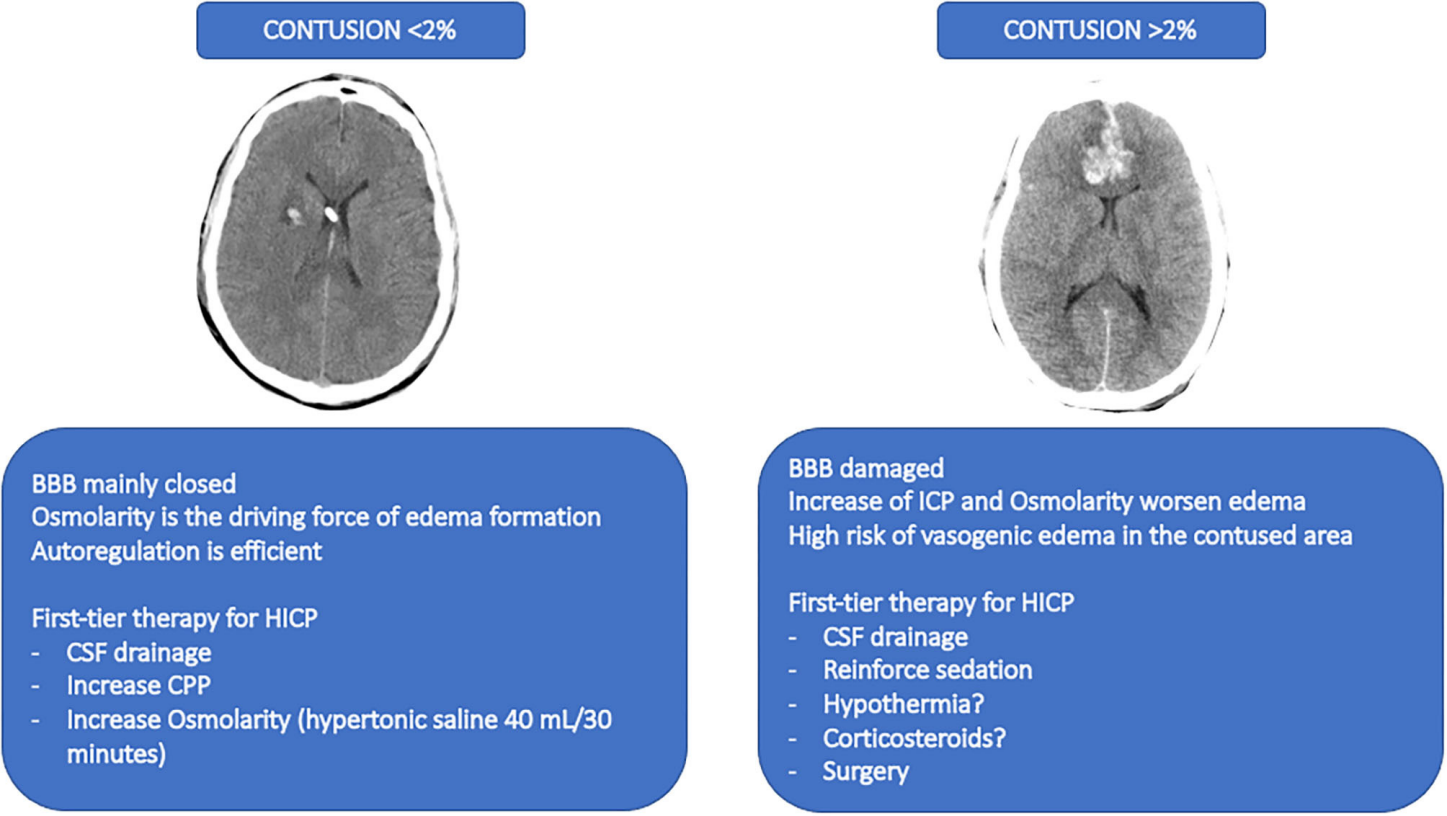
A former point-of-view for novel therapeutic approaches. The figure depicts a proposed therapeutic approach based on a former point-of-view no longer investigated and that should be reinterpreted in light of the progresses in TBI research. The image on the left represents a CT-scan with a contusion <2%. The suggested therapies for this condition are described below. The image on the right represents a CT-scan of a contusion of more than 2%. The suggested therapies are described below. BBB, blood brain barrier; HICP, intracranial hypertension; CSF, cerebral spinal fluid; CPP, cerebral perfusion pressure.
